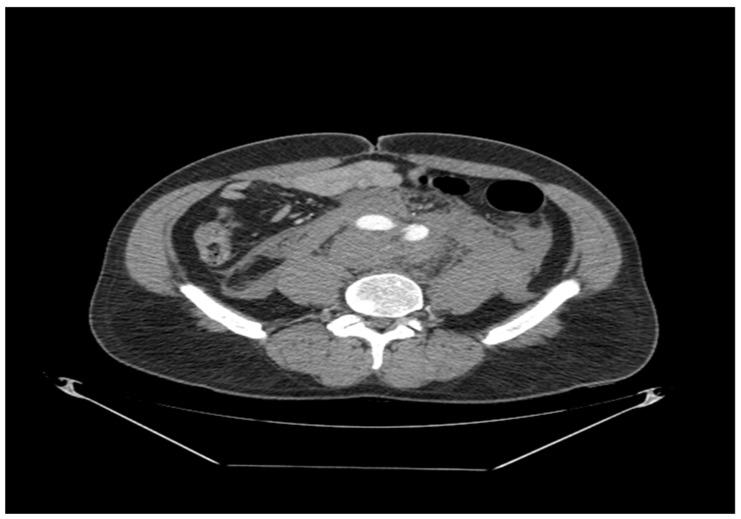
Axial CT scan demonstrating propagation of aortic dissection in left common iliac artery (diameter 28 mm).
